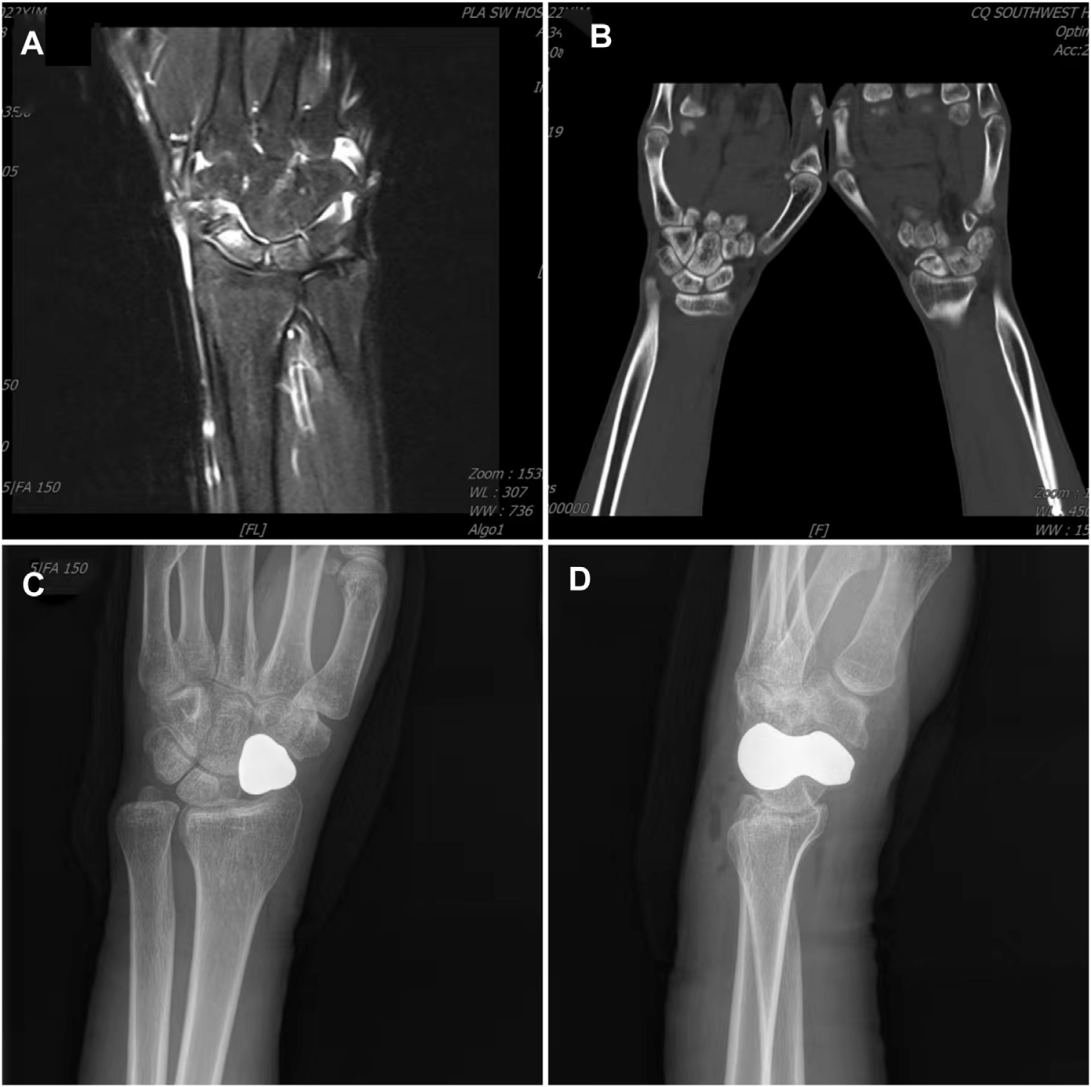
Typical case. (A) Preoperative MRI shows scaphoid osteonecrosis; (B) Preoperative CT also shows scaphoid osteonecrosis; (C) Anteroposterior radiograph of the carpal joint 2 days after surgery; (D) Lateral radiograph of the carpal joint 2 days after surgery.
